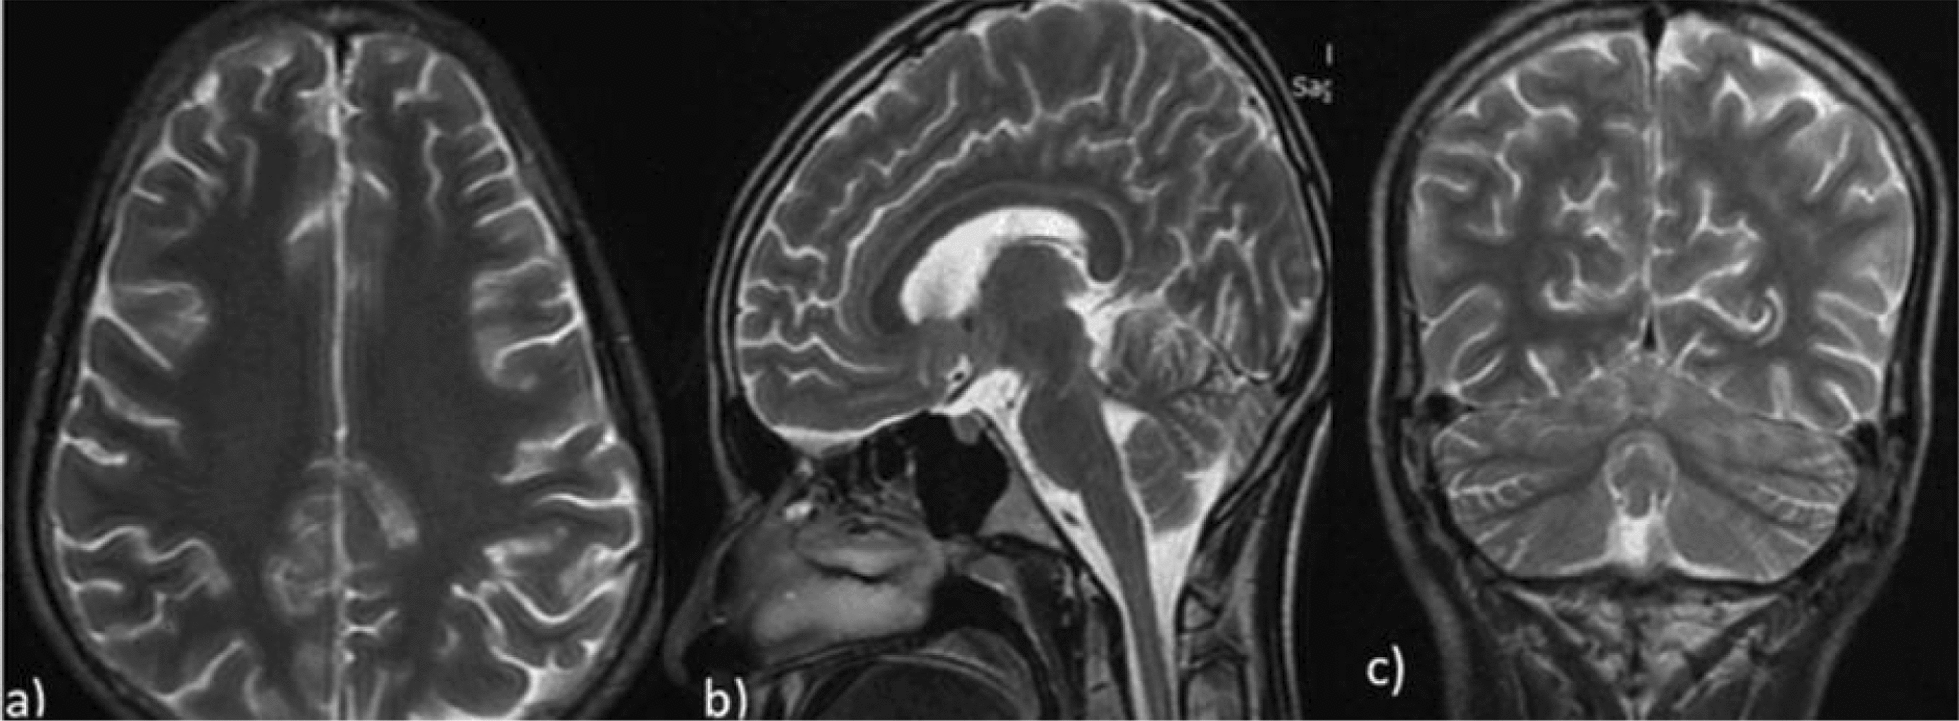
T2WI axial, sagittal, and coronal MRI shows partial resolution of lesions in the same patient after 2 weeks. Axial (image a), sagittal (image b), and coronal (image c) T2-weighted MRI images obtained after 2 weeks demonstrate partial resolution of previously noted hyperintense lesions consistent with posterior reversible encephalopathy syndrome (PRES), indicating partial radiological improvement in the same patient
